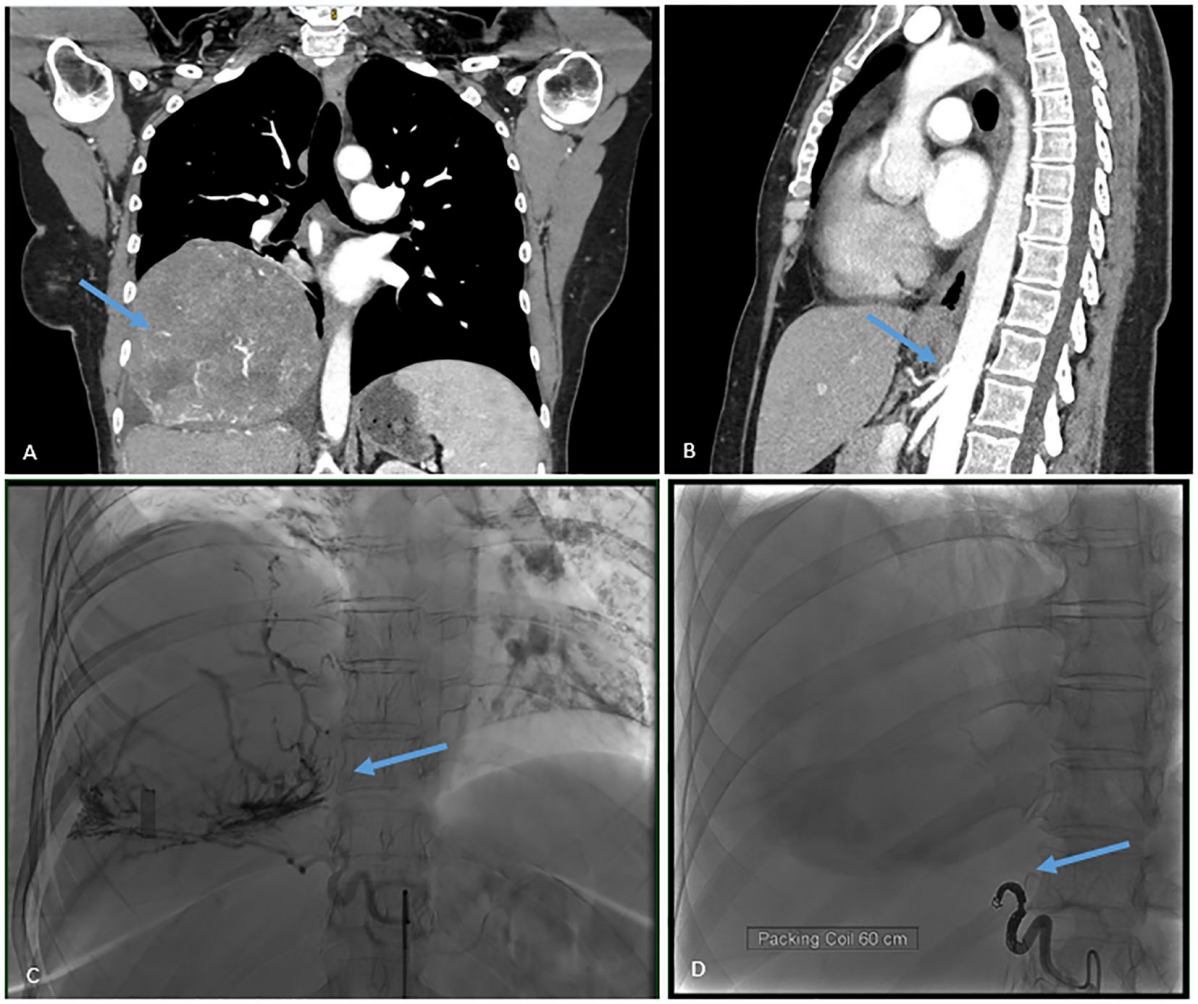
(A) CT scan showing the tumor. (B) CT scan showing the vascular pedicle arising directly from the aorta. (C) Pre-operative embolization before coiling. (D) Pre-operative embolization after coiling.
